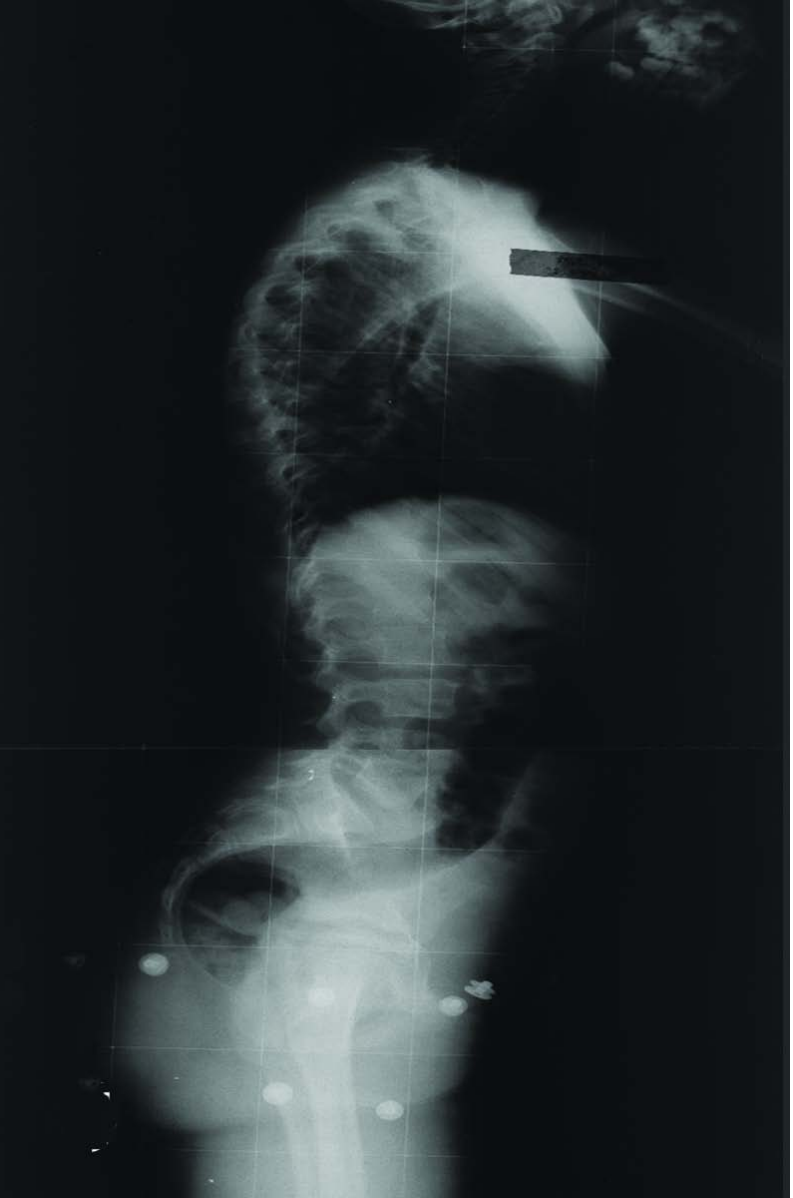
Preoperative lateral radiograph of the same patient.
